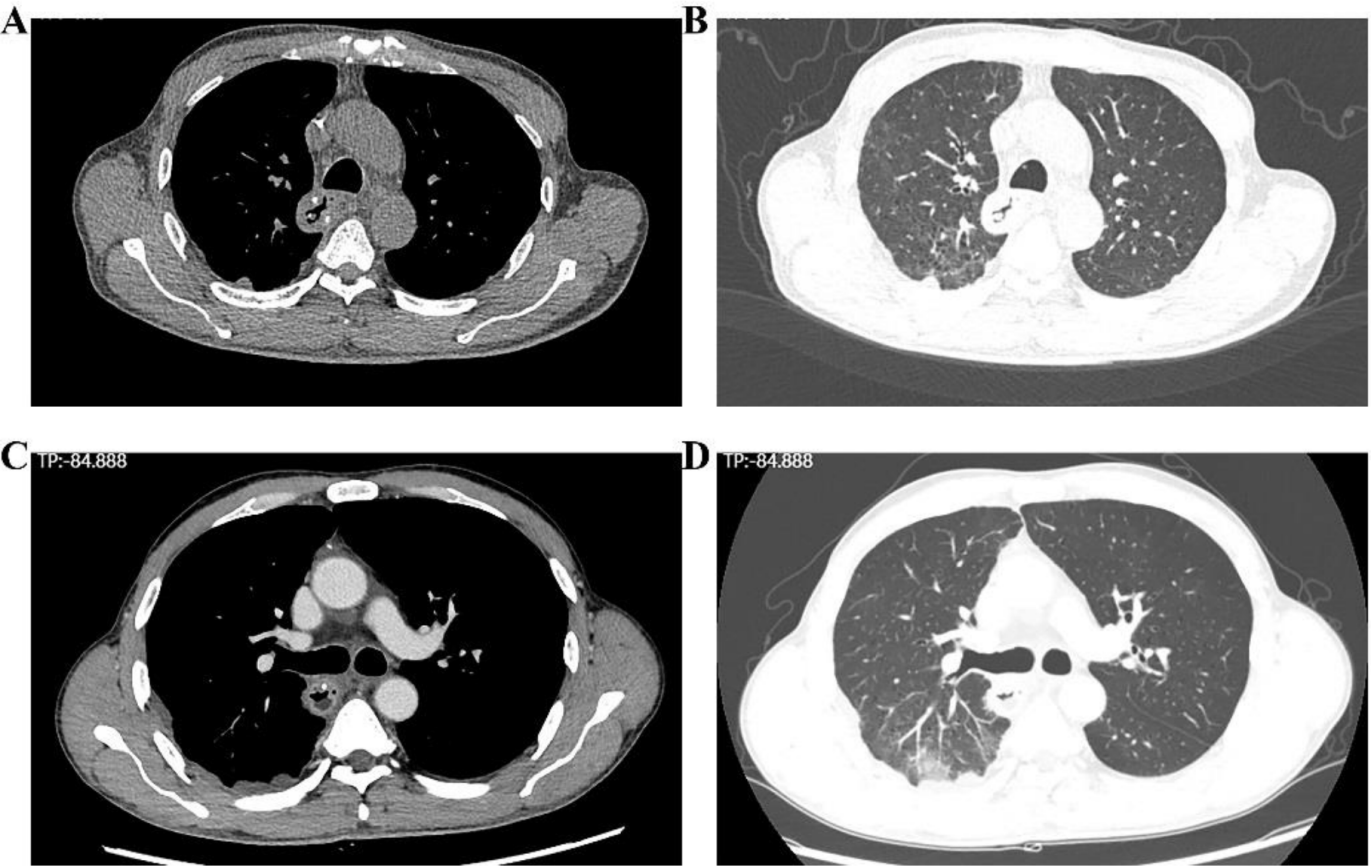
Chest CT scan showing postoperative changes in the esophagus. (A, B) The results of the chest CT scan of the patient before discharge; (C, D) The results of the chest CT scan of the patient after discharge.
